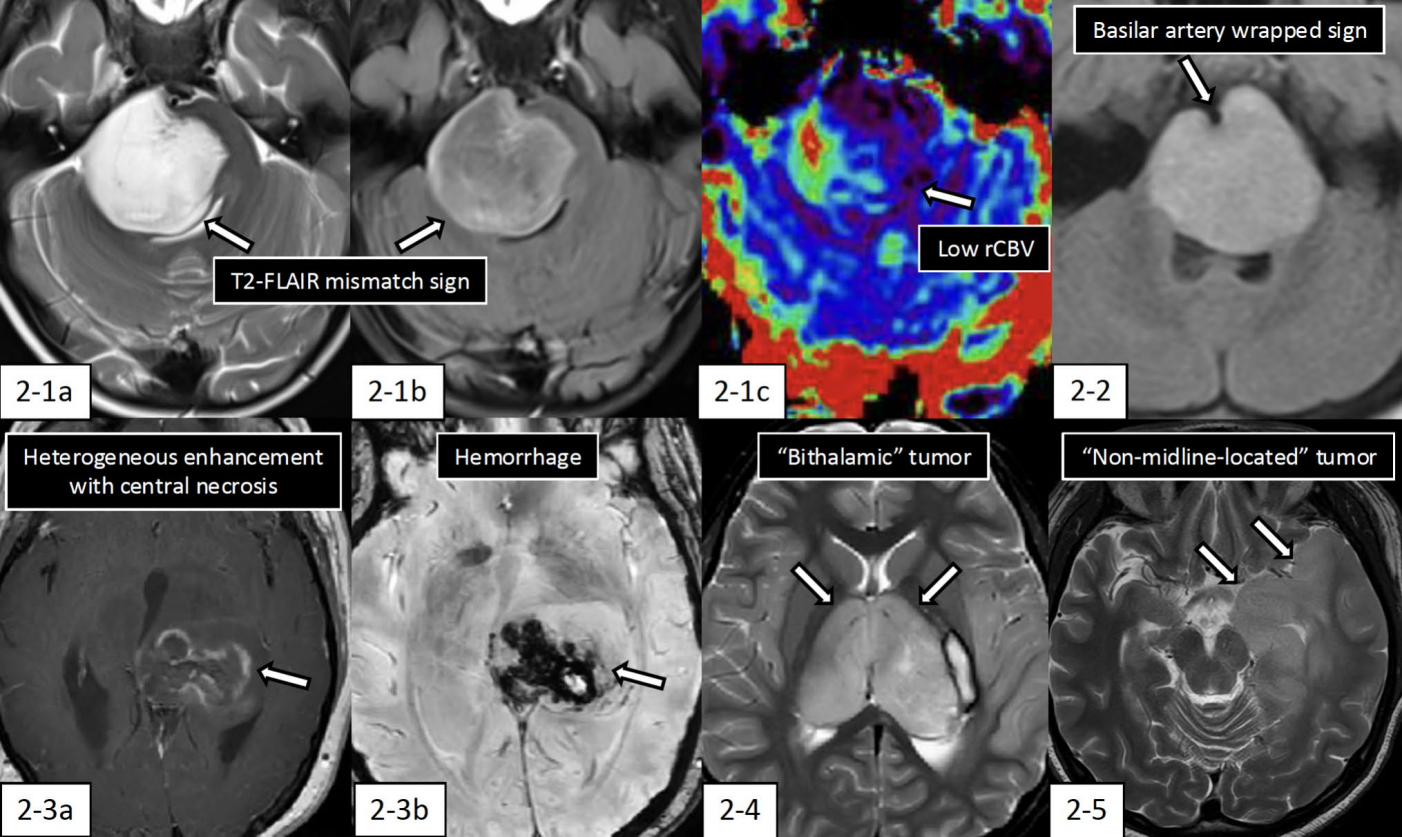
Imaging features of diffuse midline glioma, H3 K27-altered. 2-1 (4 years old, girl): Pontine tumor mass shows “T2-fluid-attenuated inversion recovery (FLAIR) mismatch sign” (2-1a and 2-1b, arrows). The relative cerebral blood volume (rCBV) shows low values in most regions of the tumor (2-1c, arrow). 2-2 (6 years old, girl): FLAIR image shows “basilar artery wrapped sign (arrow). 2-3 (36 years old, woman): The left thalamic tumor mass shows heterogeneous enhancement with central necrosis on contrast-enhanced T1-weighted imaging (2-3a, arrow) with hemorrhage shown on susceptibility-weighted imaging (2-3b, arrow). 2-4 (11 years old, girl): The “bithalamic” tumor mass is observed on fat-suppressed T2-weighted imaging (arrows). This tumor harbored H3.1K27M-mutant and an EGFR A289V mutation with an allele fraction of 56%. 2-5 (25 years old, woman): “Non-midline-located” diffuse glioma with H3K27M-mutant. The tumor mass mainly involves the left temporal lobe (arrows)
